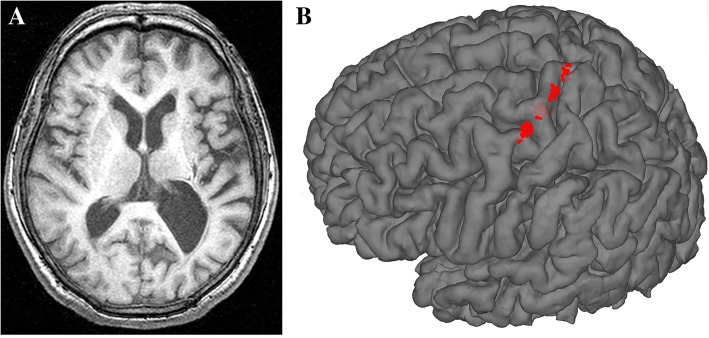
Neuroimaging data from patient 2 (MWH, female, 74 years old, left thalamic hemorrhage 20 years ago); a Presurgical T1-weighted MRI (left ventricle enlargement); b Three-dimensional reconstruction of electrode placement (left precentral gyrus)
